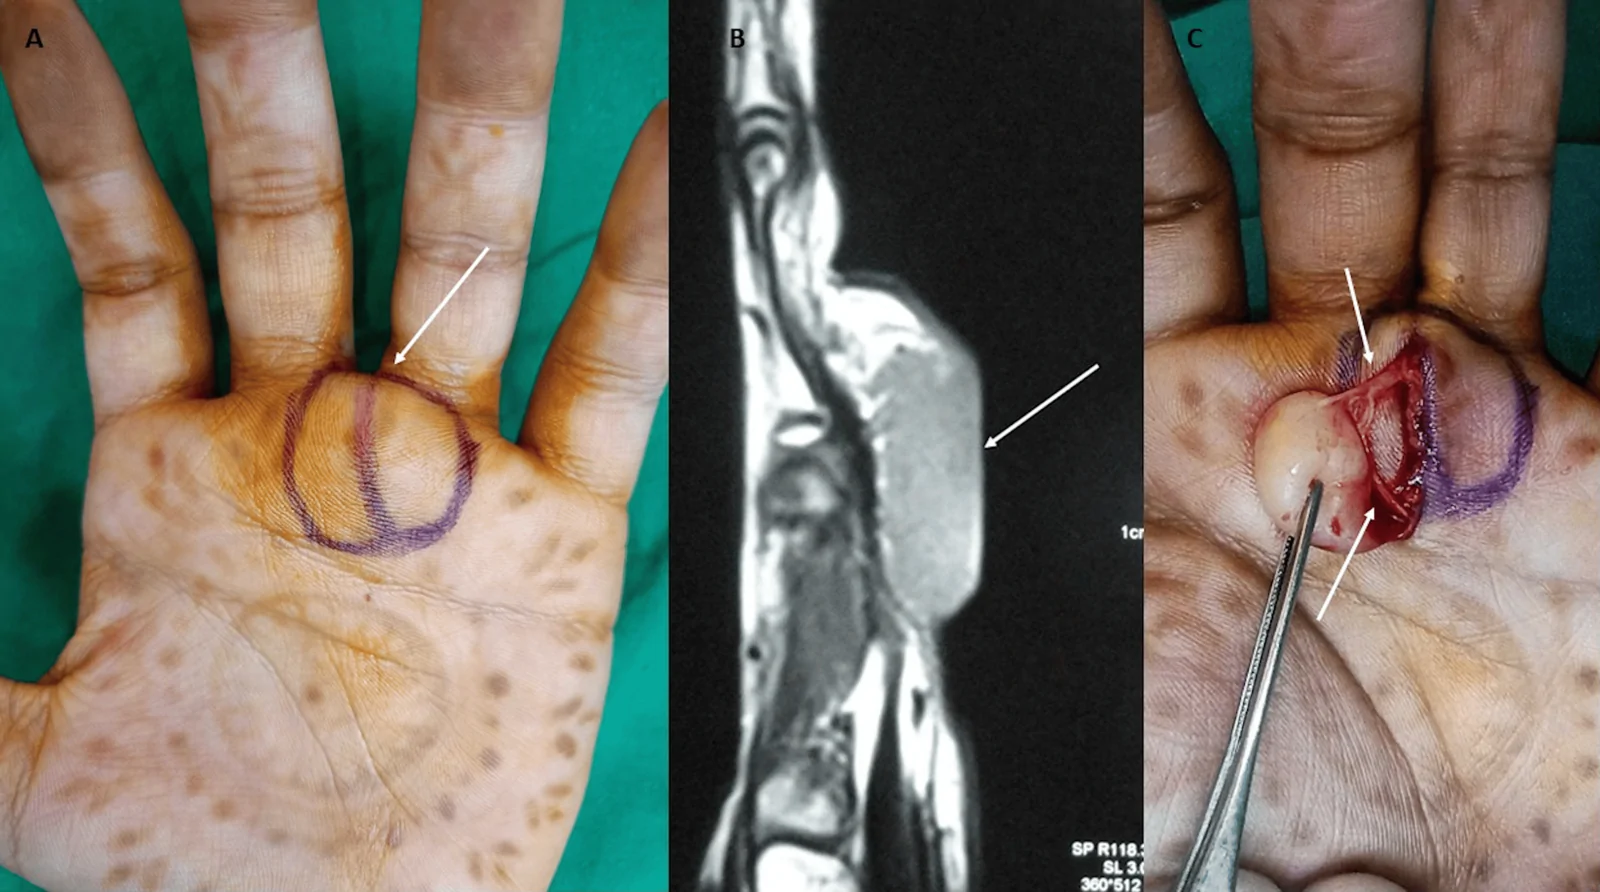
Clinical, radiological, and intraoperative findings of the palmar swelling at the base of the ring finger (A) Swelling at the base of the ring finger encroaching into the web space. (B) MRI showed a well-encapsulated iso-to-hypointense (T1) lesion with incomplete fat suppression in the palmar region at the base of the ring finger. (C) Intraoperative view showing the origin of a tumor from a branch of the digital nerve MRI: magnetic resonance imaging
